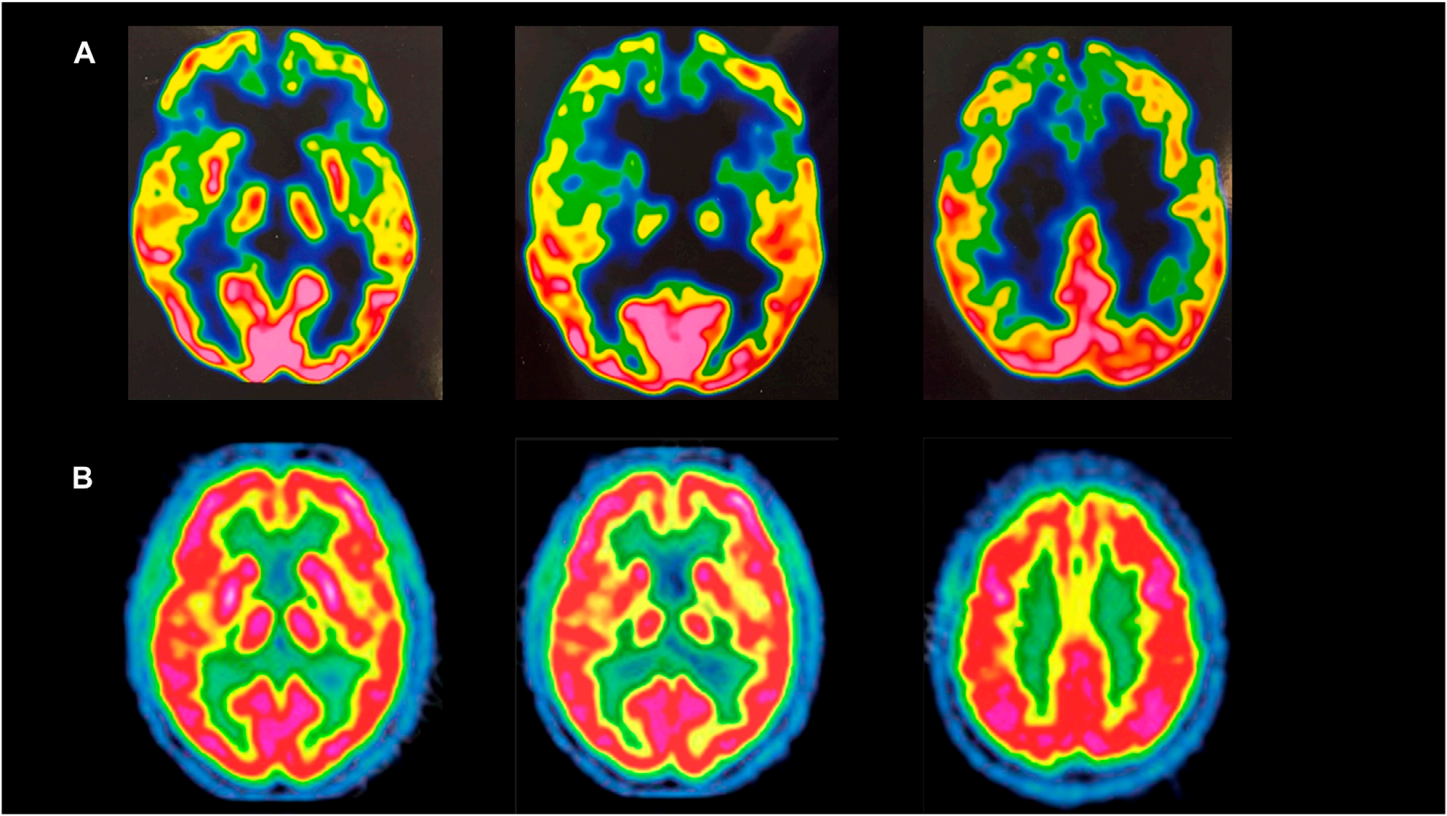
(A) Brain 18-FDG PET of the mother, showing severe hypometabolism in the fronto-temporal brain cortex bilaterally. (B) Brain 18-FDG PET of a healthy subject.
